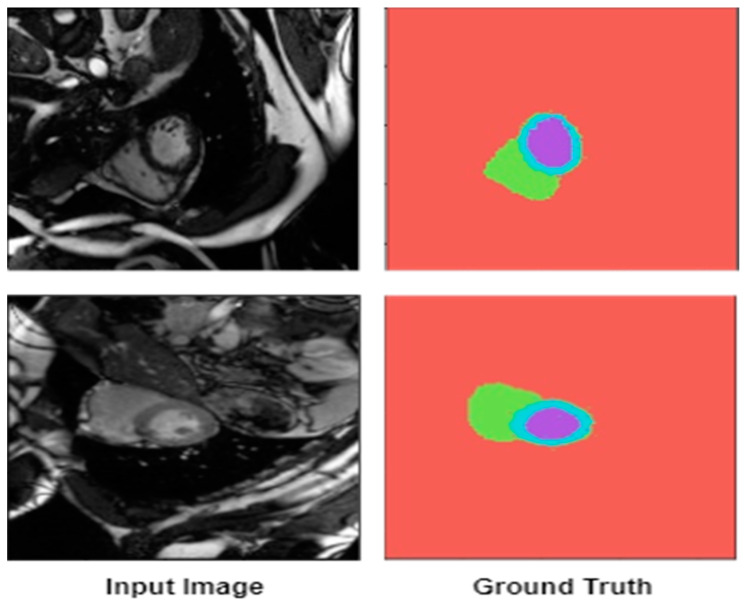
Sample of cardiac magnetic resonance images from the ACDC dataset.
